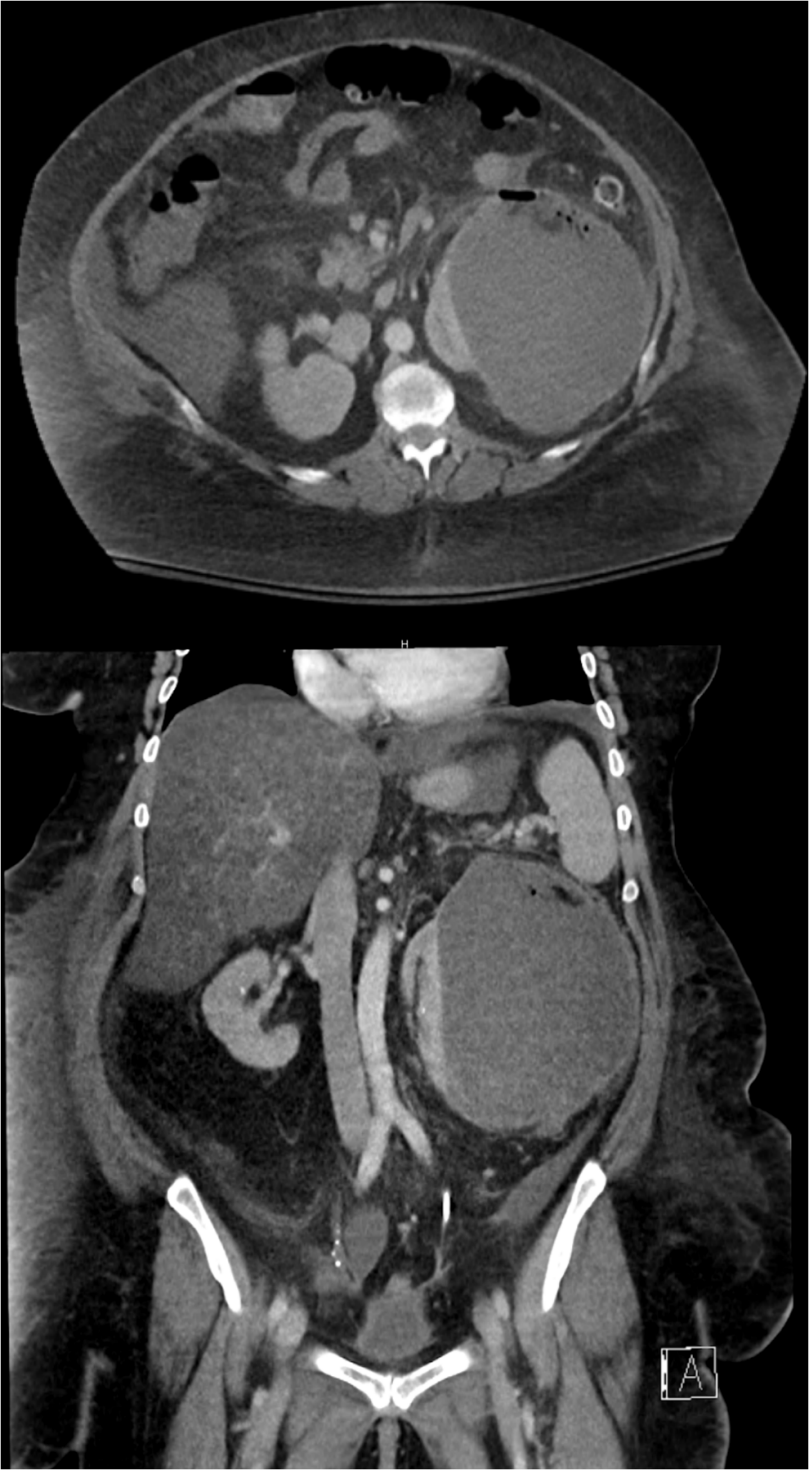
CT scan showing large left perinephric fluid collection containing locules of gas with surrounding inflammatory perinephric fat stranding.
